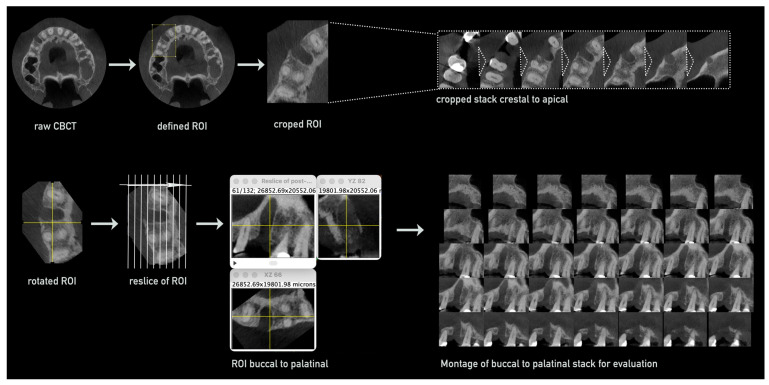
Semi-automated CBCT processing for volume and density evaluation. The raw CBCT data were imported, followed by the definition of the region of interest (ROI) and cropping to isolate the area including the treated socket. The resulting stack, encompassing the entire region from the crestal to the apical aspect, was resliced from buccal to palatal/lingual. For the final evaluation, a montage of the resliced buccal-to-palatal/lingual stack was created.
